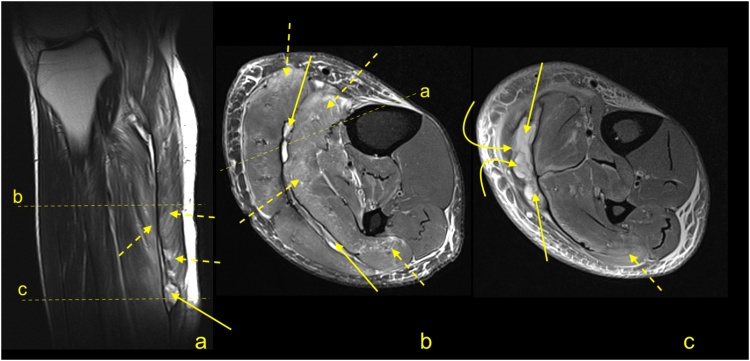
A 25-year-old man who played football and at the end of training felt acute pain in the calf. MRI (a–c) showed a hematoma separating the aponeuroses of the gastrocnemius and soleus muscles (straight arrow) and extensive muscle edema in the soleus and gastrocnemius muscles (dashed arrow). The defect at the musculotendinous junction of the medial head of the gastrocnemius is visible (curved arrow). A- PD- weighted, b and c- PD- weighted with fat suppression.
